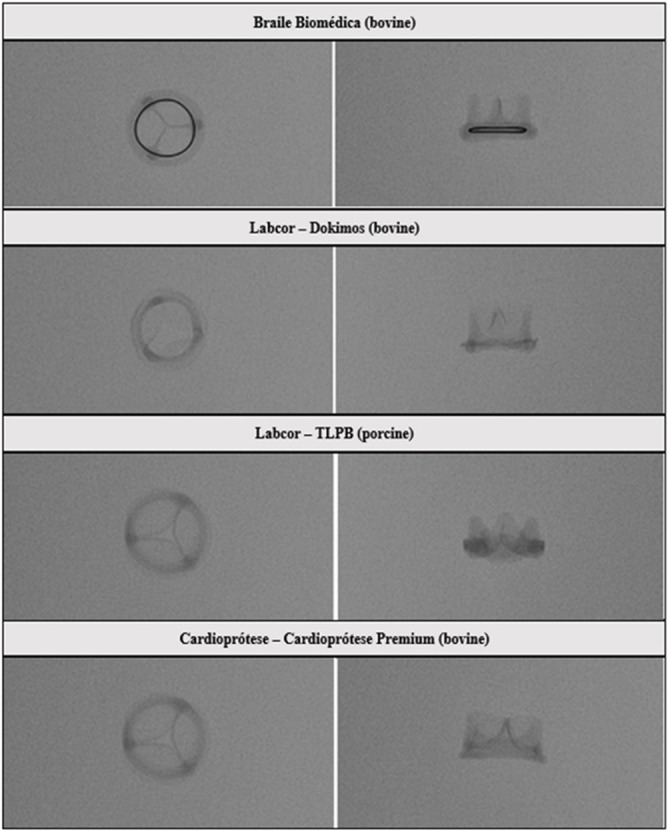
Radiography of the prostheses used in the test. Superior - inferior and lateral projections.
